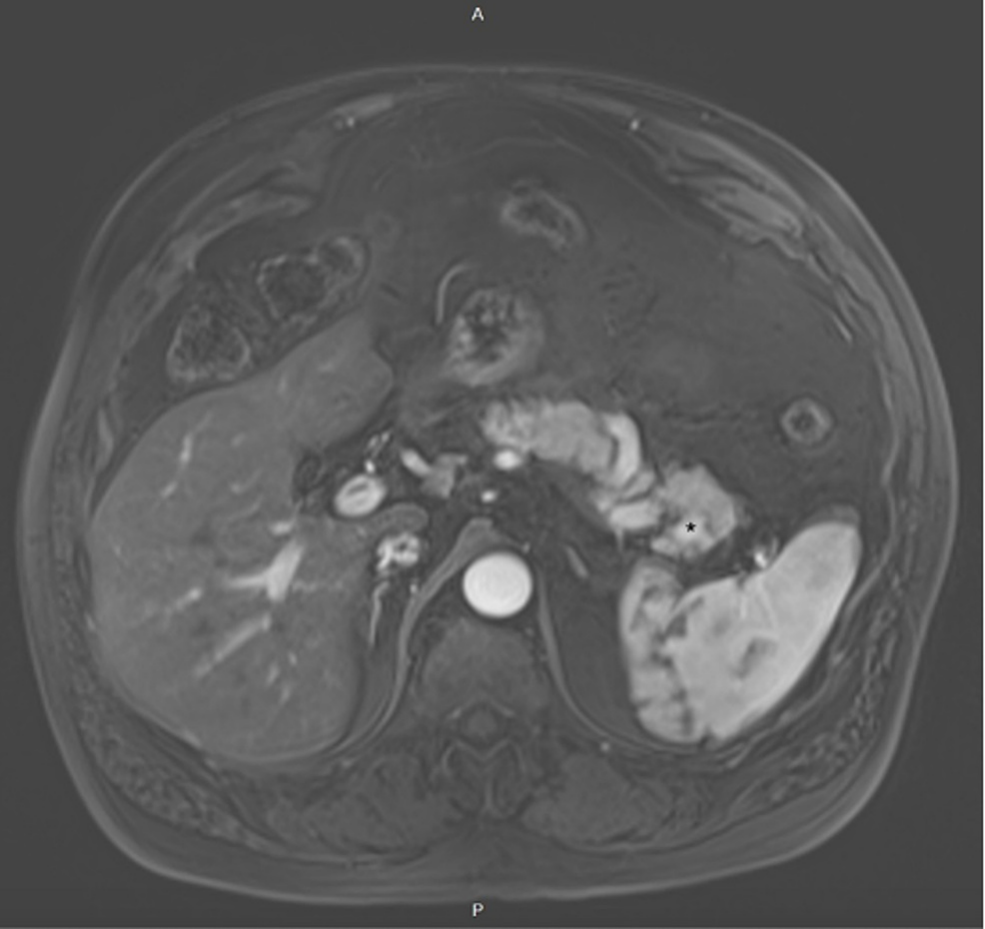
MRI, axial T1 fat-saturated post-contrast image with a pancreatic tumor. Pancreatic tumor marked (*)
